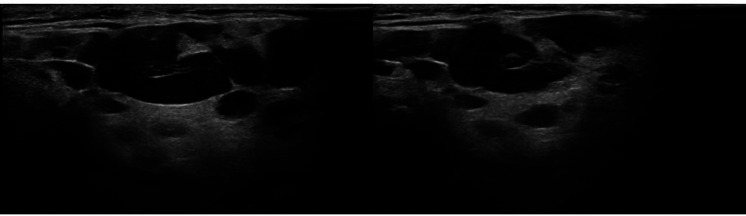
Ultrasound examination shows normal-sized bilateral parotid glands containing multiple bilateral solid hypoechoic nodular lesions.
